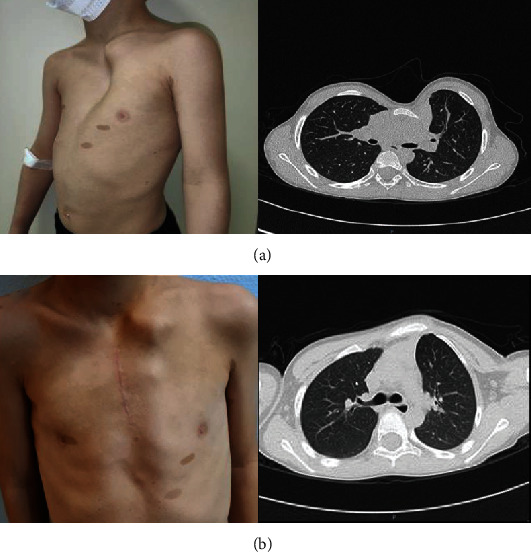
Clinical and imaging findings. Clinical photographs and axial CT scans of the chest of the reported individual before (a) and after (b) surgical intervention. The patient had a severe deformity of the anterior chest wall and some typical cafè-au-lait macules (a). The PE deformity consisted of a severe dorsal deviation of the sternal manubrium and costal cartilage, as shown by the preoperative CT scan (a). After surgery, a significant correction of the PE deformity was achieved. This result could be appreciated at the clinical exam and was confirmed by follow-up CT scan (b).
